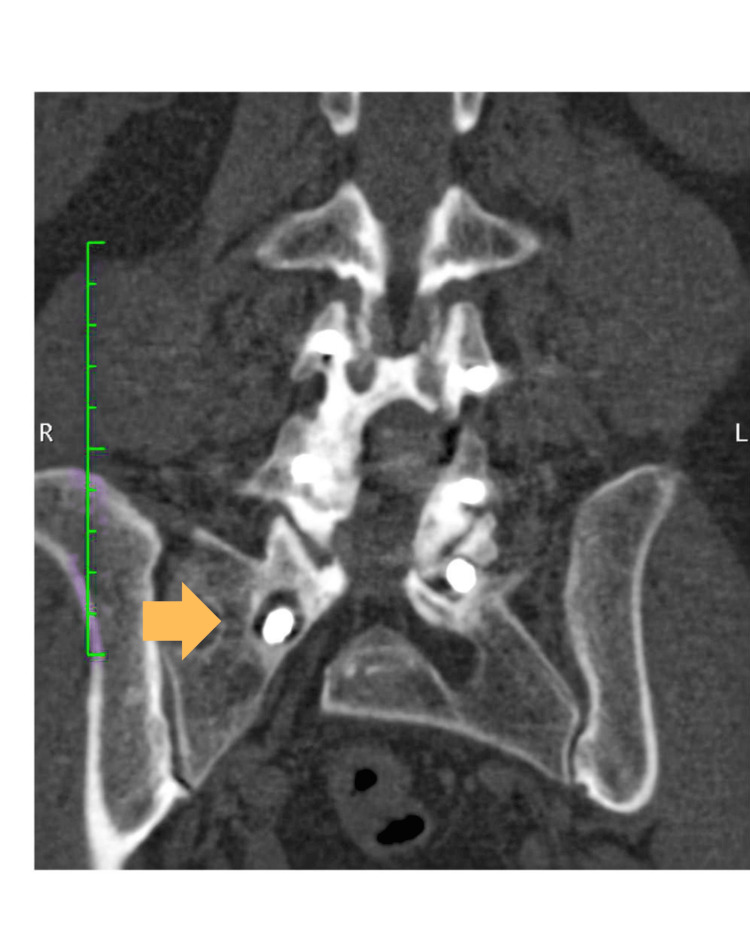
Computed tomography sagittal section of the lumbar spine with right L4–L5 facet fusion, with void sign at L5–S1
